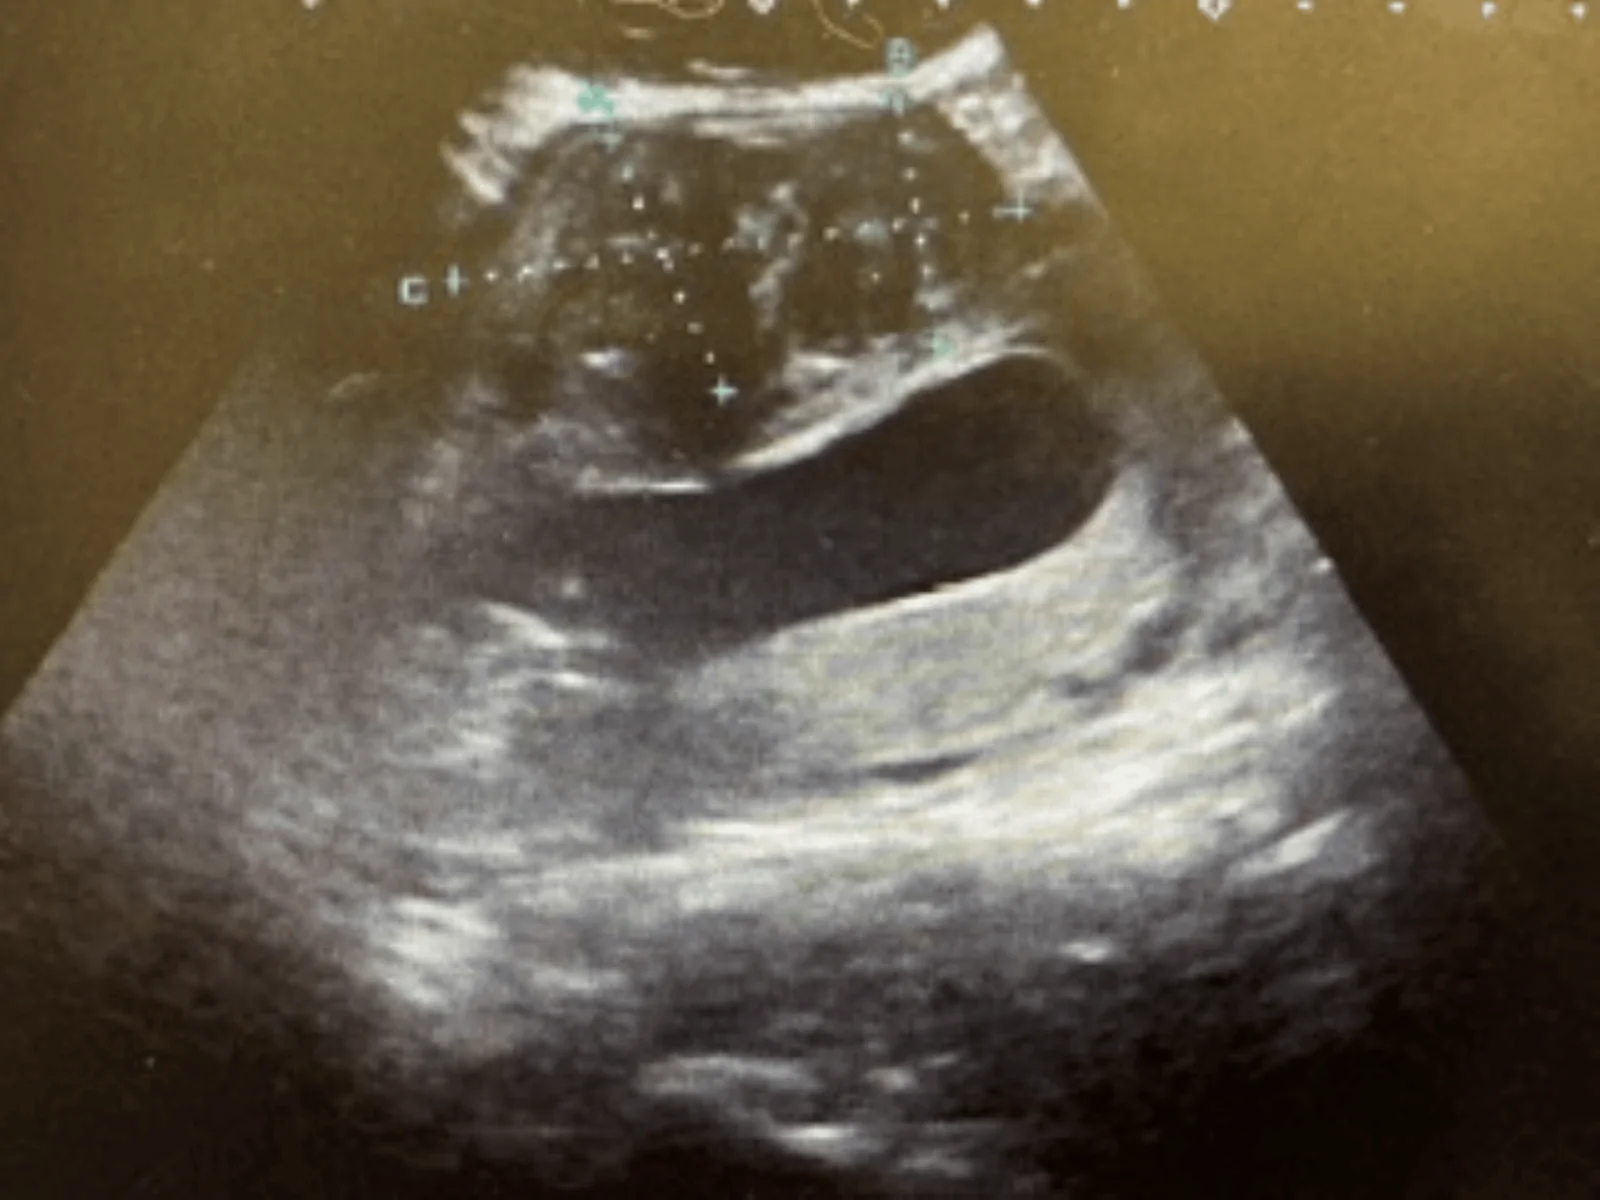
Anterior wall fibroid seen on ultrasound.
